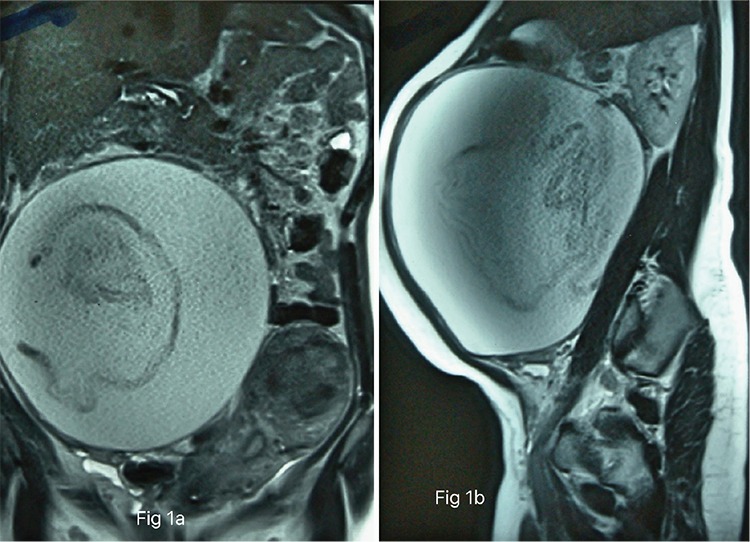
T1-weighted magnetic resonance imaging images (a) Coronal section (b) Sagittal section
